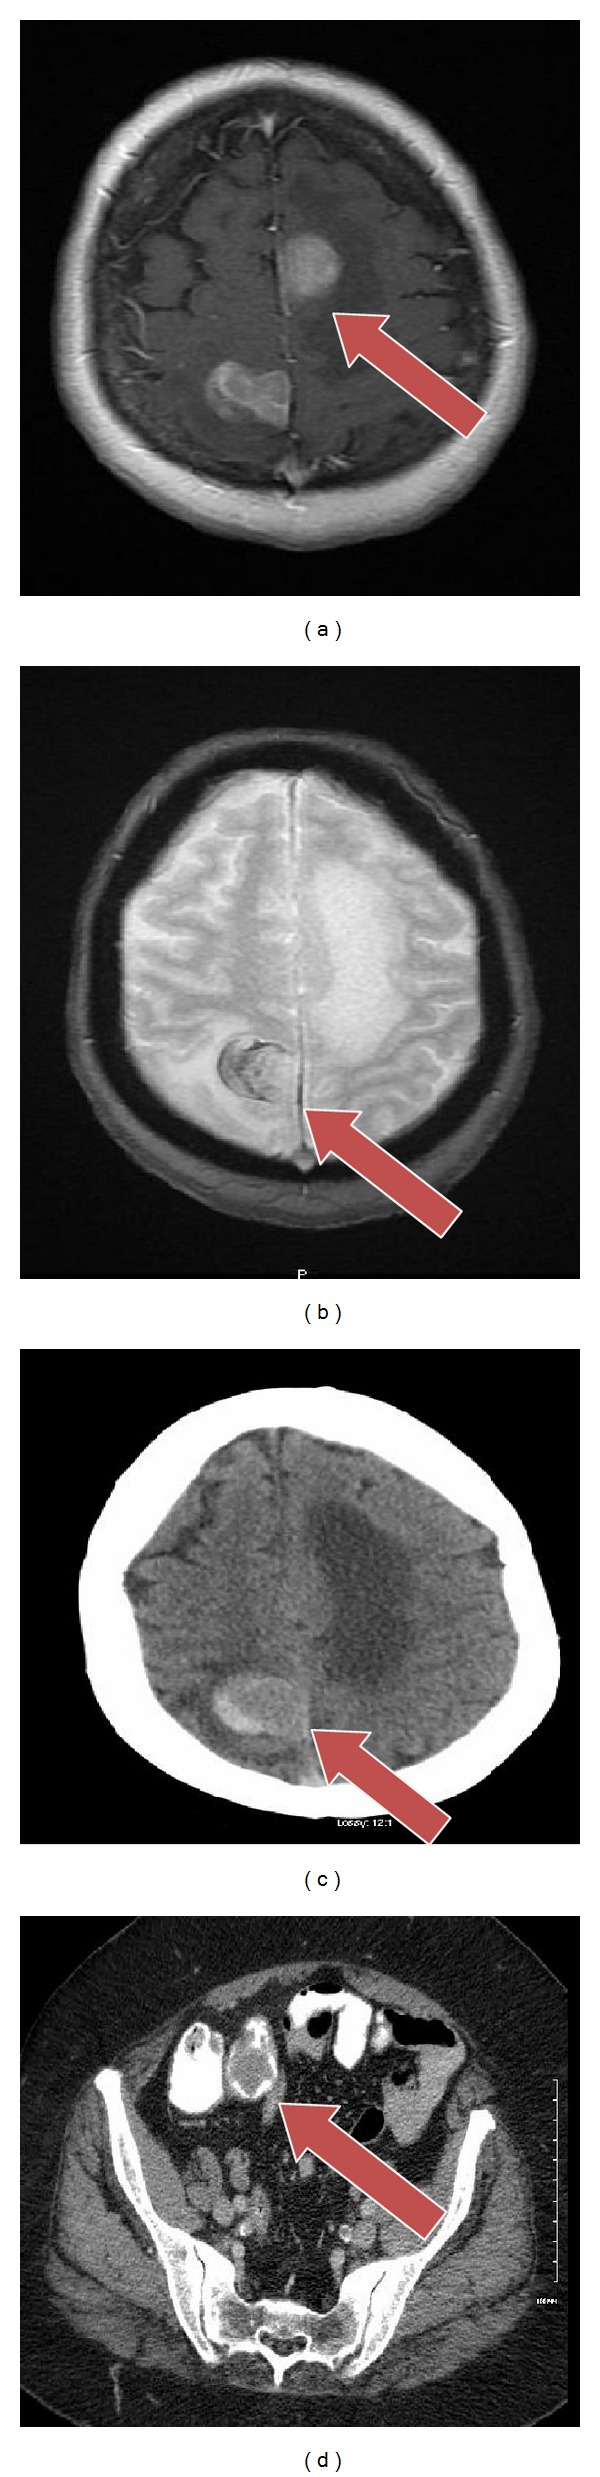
(a), (b), (c) Arrows showing MRI of brain with and without gadolinium with metastatic lesions in parietal and occipital region. (d) Showing CT scan of abdomen and pelvis with filling defect in terminal ileum.
